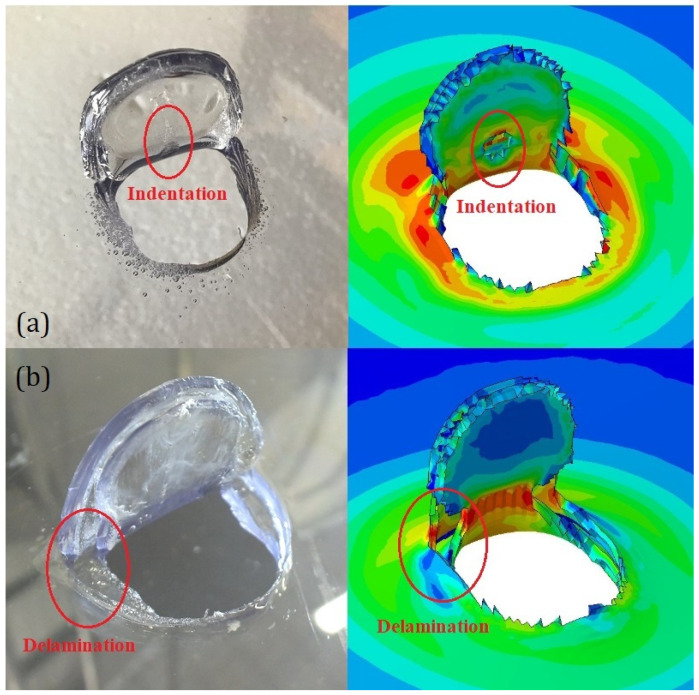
Comparison between experimental and numerical indentation morphologies for (a) an uncontained DWI test on PC and (b) an uncontained DWI test on LPC. The numerical contour show the Von Mises stress.
